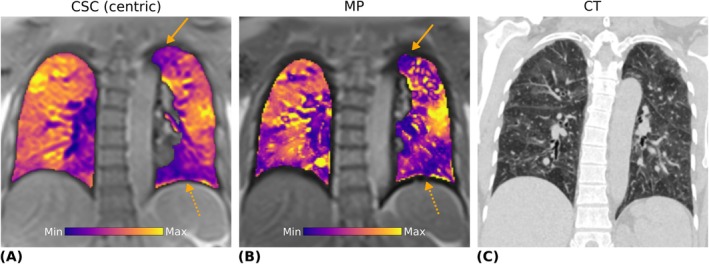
52‐year‐old female patient with T2‐low asthma. The CSC (A) and MP (B) ventilation maps of 10 mm slice thickness indicate peripheral functional impairment in the lower lobe of the left lung (dashed arrow) as well as in the apical region of the left lung (solid arrow). The corresponding CT image shows only mild inhomogeneities of lung parenchyma (C).
